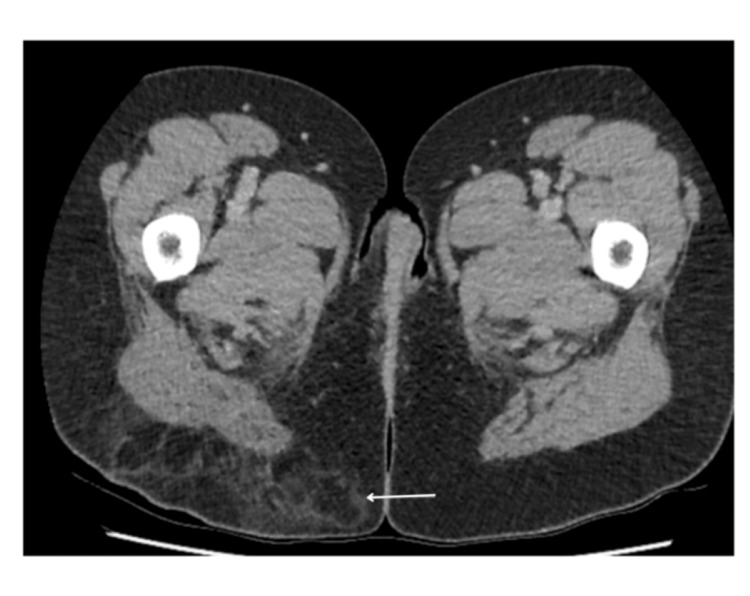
Axial post-contrast venous phase pelvic CT scan. Progression of appearances within the subcutaneous fat overlying the right buttock showing marked inflammatory fat stranding (arrow)
